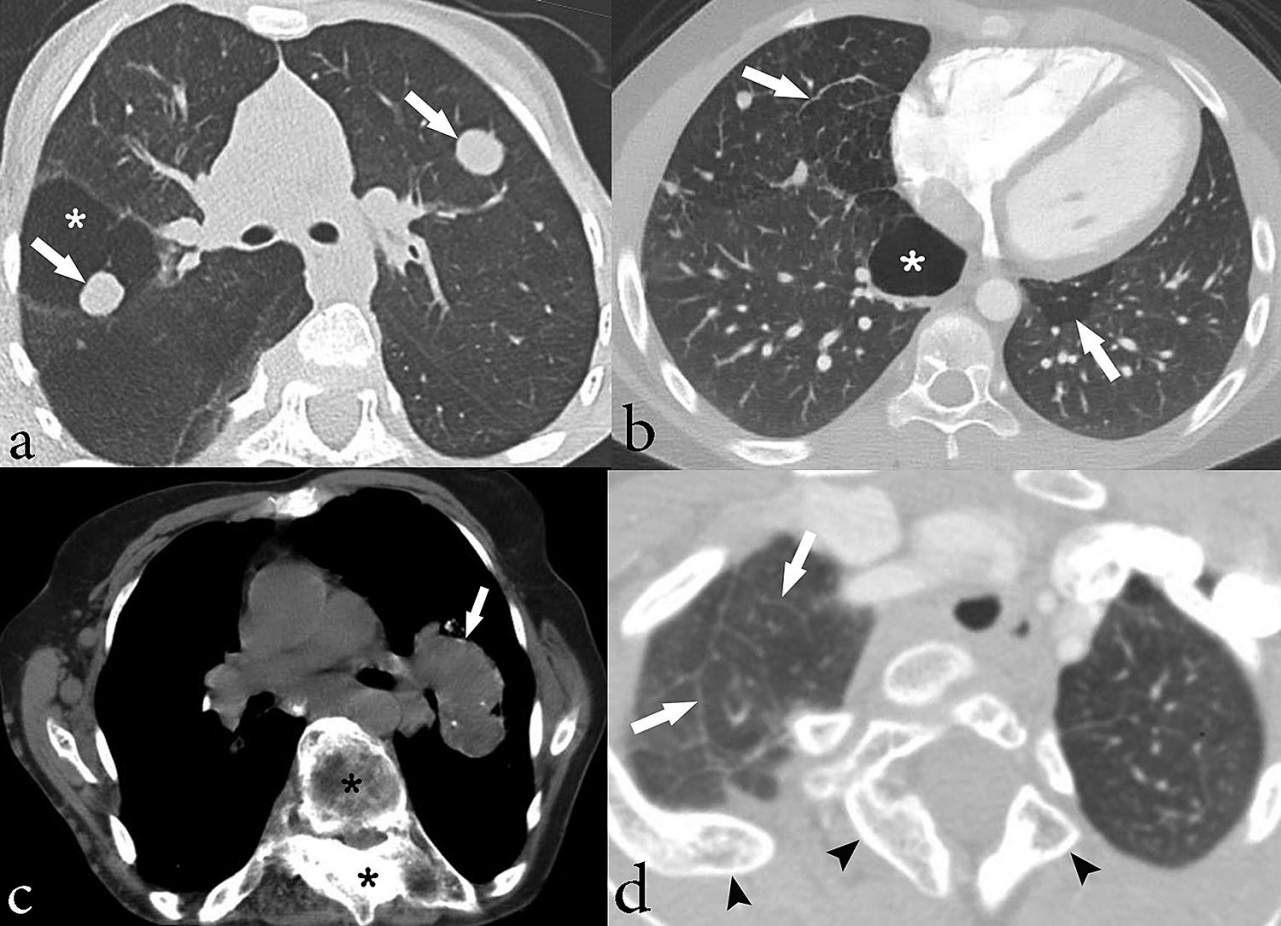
Chest CT images showing lung parenchymal involvement in individuals with Proteus syndrome. Axial CT images in lung windows show (a) nodules in the bilateral upper lobes measuring up to 17 mm in size (arrows) in a 9-year-old male. An area of cystic change is also noted in the right upper lobe (asterisk); (b) areas of hyperlucent lung parenchyma within the right middle lobe and left lower lobe (arrows) in a 10-year-old male. There is also a cyst in the right lower lobe (asterisk) that abuts a curvilinear band of scarring posteriorly. (c) Axial CT image in soft tissue windows shows a lobulated mass in the perihilar left upper lobe (arrow) in a 38-year-old female nonsmoker, which was found to be primary lung adenocarcinoma on pathology. Overgrowth of the vertebral body and posterior elements of the spine are also noted (asterisks). (d) Axial CT image in lung windows shows subtle interlobular septal thickening (arrows) in the right upper lobe in a 14-year-old female. Skeletal overgrowth involving the posterior spinous elements and right posterior rib are also noted (arrowheads).
